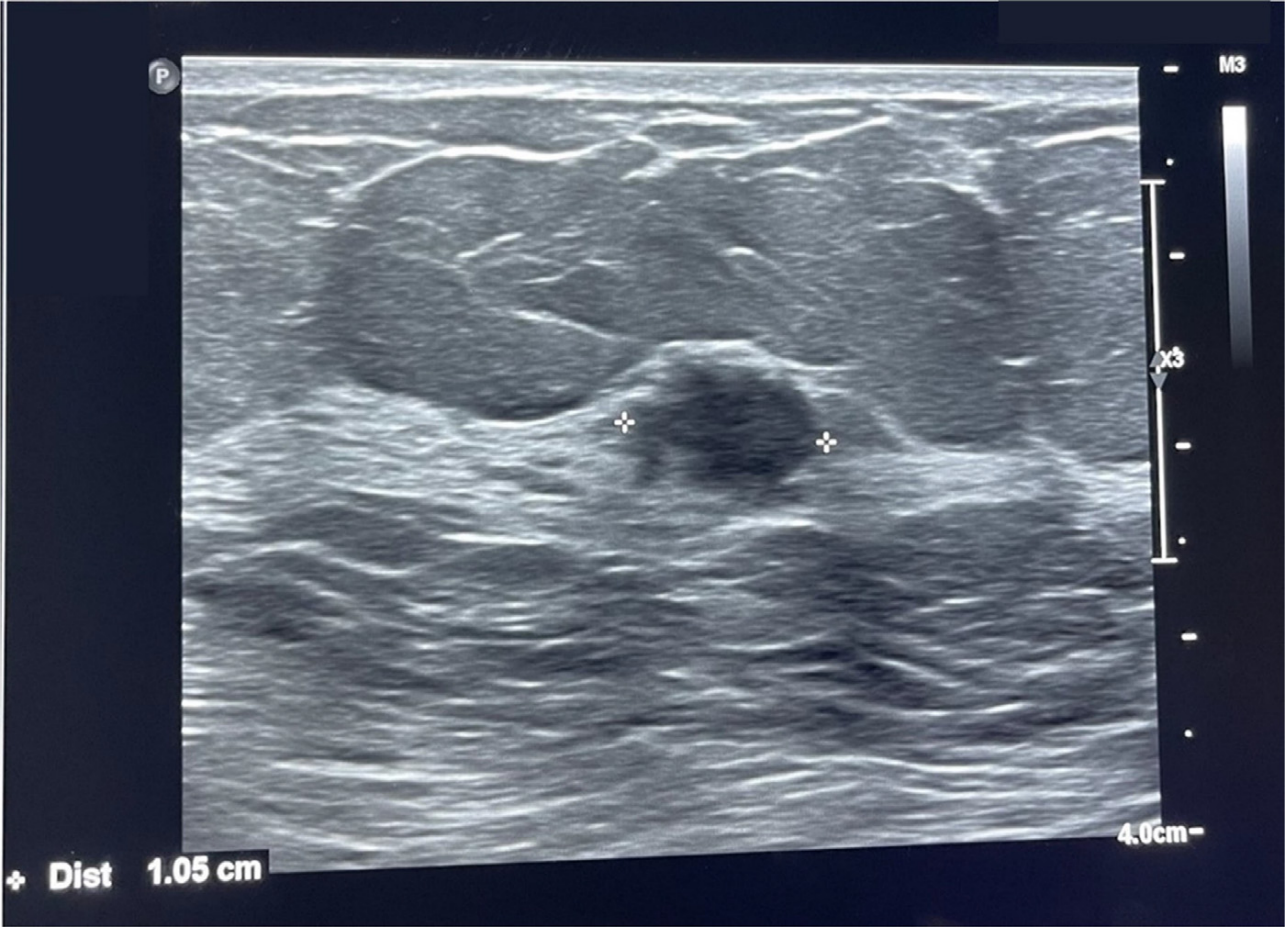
Ultrasound showed an oval-shaped formation with heterogeneous hypoechogenicity.
